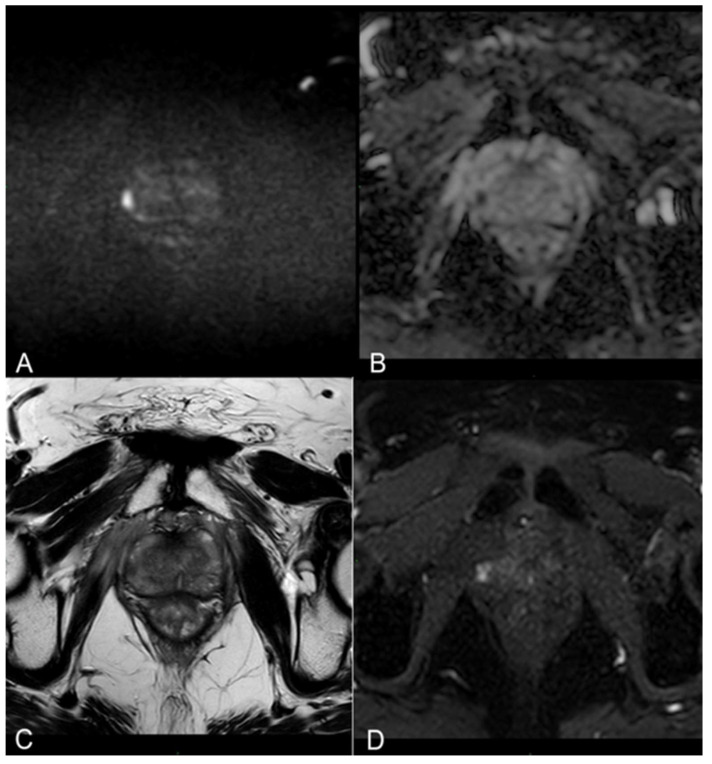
(A,B) Diffusion-weighted imaging (DWI) b-value 2500 s/mm^2^ and ADC map on axial plane showing focal marked hyperintensity on high b-value DWI sequences corresponding to a hypointensity on the ADC map (PI-RADS 4). (C) T2-weighted TSE sequence on axial plane showing a hypointense lesion in the peripheral zone of the right middle portion of the gland. A curvilinear contact surface with the prostate capsule is demonstrated without any sign of capsule invasion. (D) Dynamic Contrast-Enhanced Imaging (DCE) with T1-weighted Dixon sequence with fat suppression on axial plane: early and significant focal enhancement in the peripheral zone of the right mid-portion of the gland.
